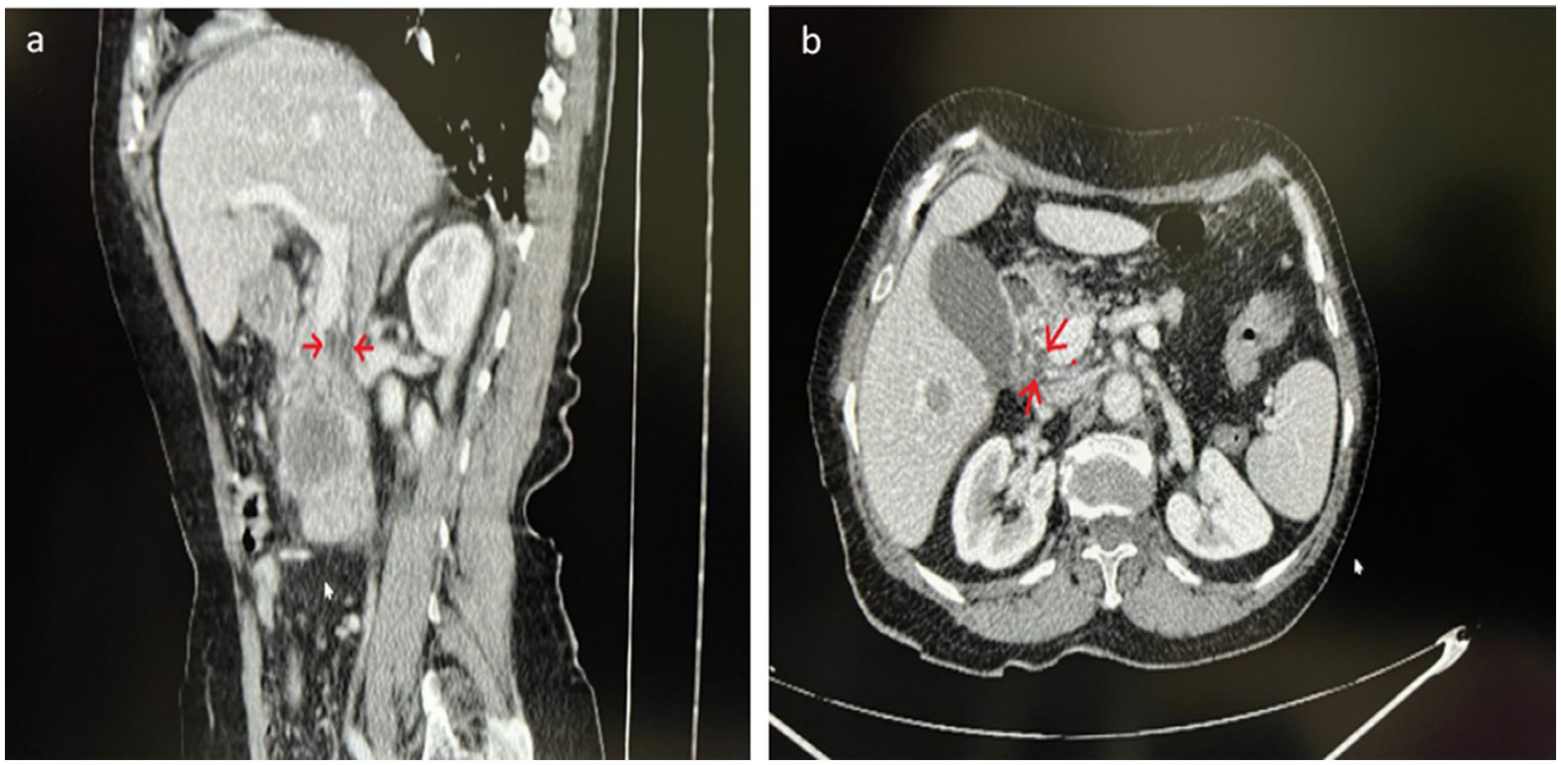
(a) Sagittal vision and (b) axial vision section of the dynamic CT images shows that the septum within the common bile duct. The septum in the common bile duct is seen in the areas marked with arrows.
